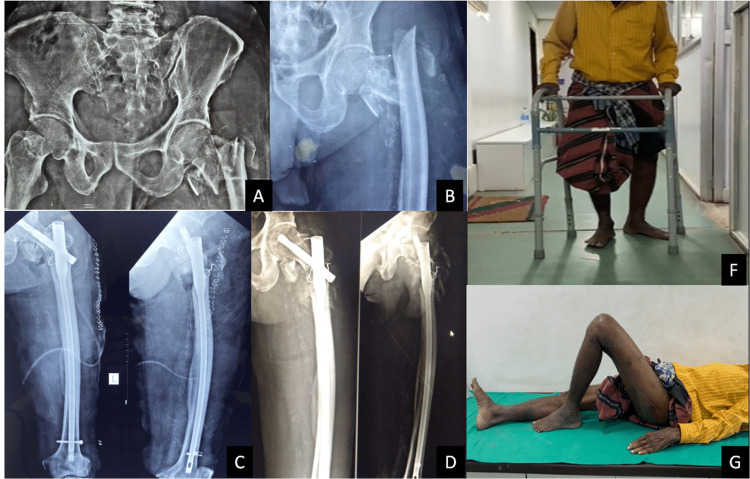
Preoperative radiographs (A, B), immediate postoperative radiograph (C), showing callus formation at six weeks (D), and six weeks of follow-up (E, F).
